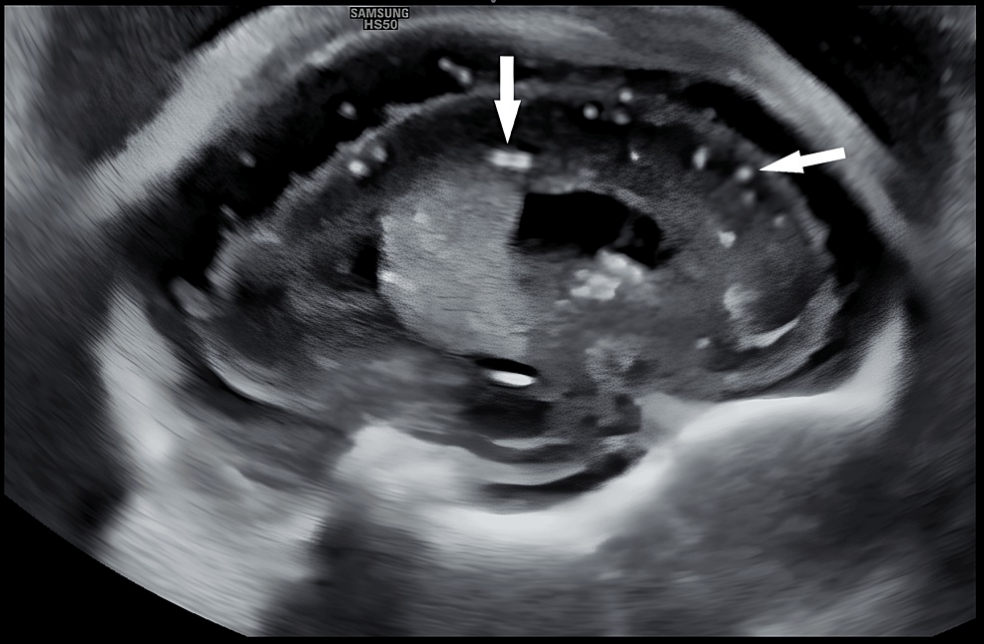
Parasagittal neuro-sonogram Echogenic foci in the cerebrum and the periventricular region as shown by white arrows.
